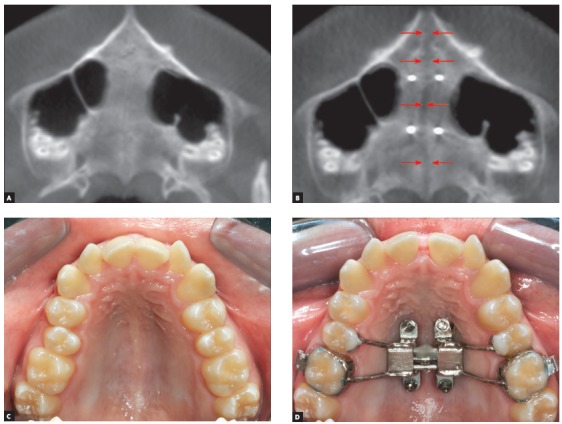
A, B) CBCT images on the axial section, before and after MARPE. Red arrows indicate the opening of the midpalatal suture. C, D) Clinical photographs, in occlusal view, before and after MARPE, respectively.
